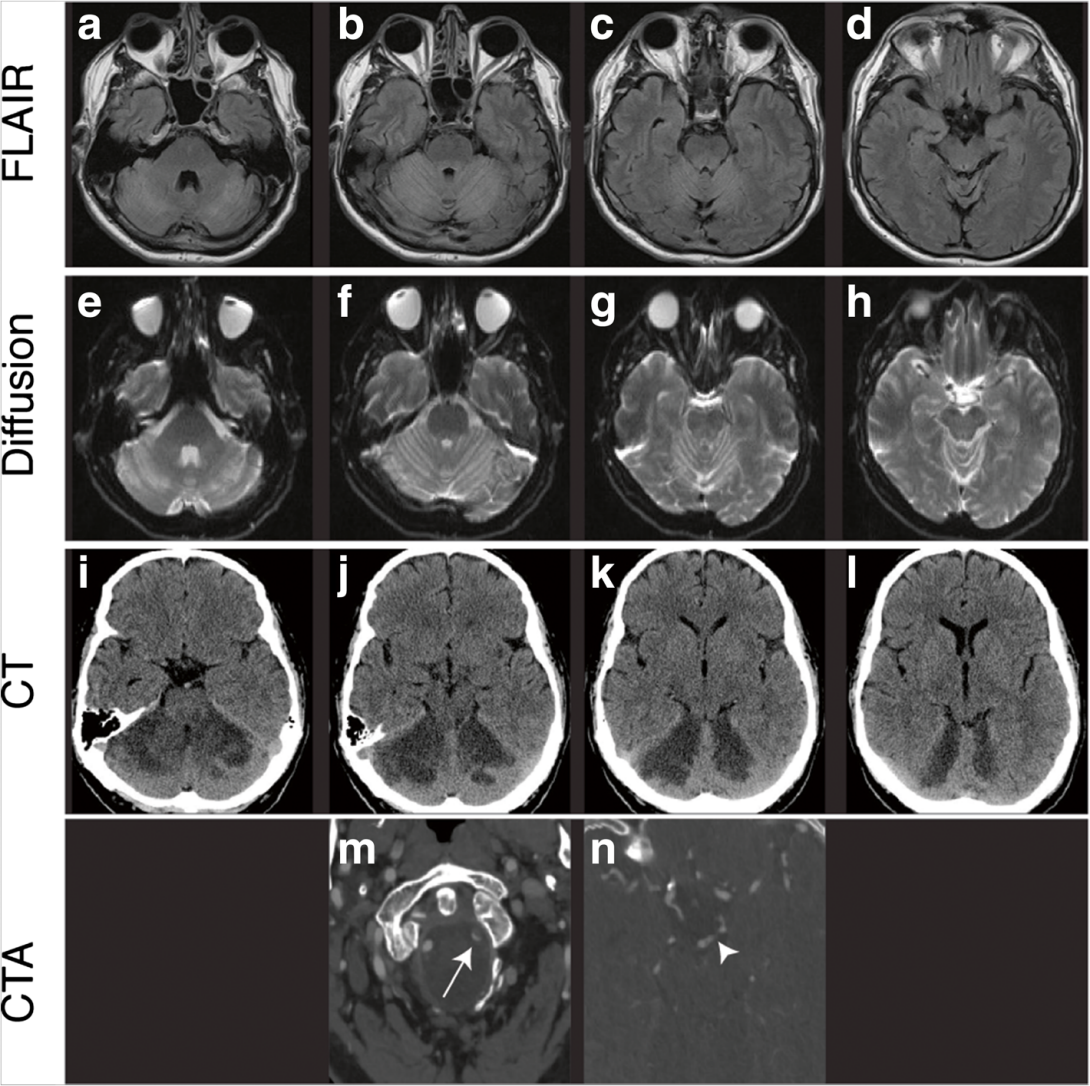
Axial 1.5 T MR, CT and CTA images demonstrate bilateral SCA infarct and left vertebral artery dissection. a–d Sequential axial FLAIR MR images demonstrate bilateral cerebellar hemisphere hyperintensity superiorly with relative sparing inferiorly (not shown) and involvement of the colliculi in the midbrain and the superior cerebellar peduncles. e–h Sequential axial diffusion MR images demonstrate restricted diffusion in both cerebellar hemispheres in the same distribution of the FLAIR images. i–l Sequential axial non-contrast CT images demonstrate patchy areas of hypoattenuation bilaterally in the superior aspects of the cerebellar hemispheres. m–n Select axial CTA images demonstrate decreased caliber of left vertebral artery with arterial lumen filling defect (arrow). As well a filling defect is present in the basilar terminus extending to the P1 segment of the left PCA (arrowhead). *MR* magnetic resonance, *FLAIR* fluid-attenuated inversion recovery, *CT* computer tomography, *CTA* computer tomography angiography, *SCA* superior cerebellar artery, *PCA* posterior cerebral artery
